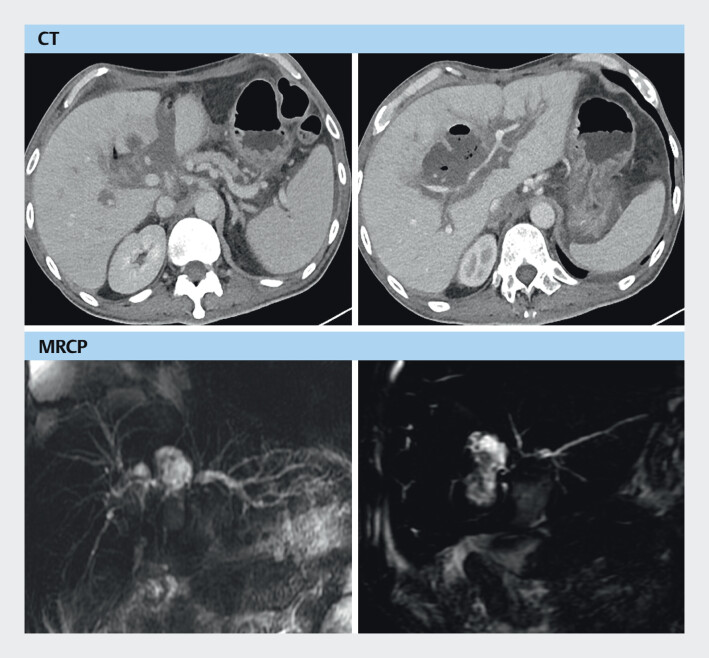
Abdominal enhanced computed tomography (CT) and magnetic resonance cholangiopancreatography (MRCP) showed post-transplant cholangiopathy with bile lake formation.
